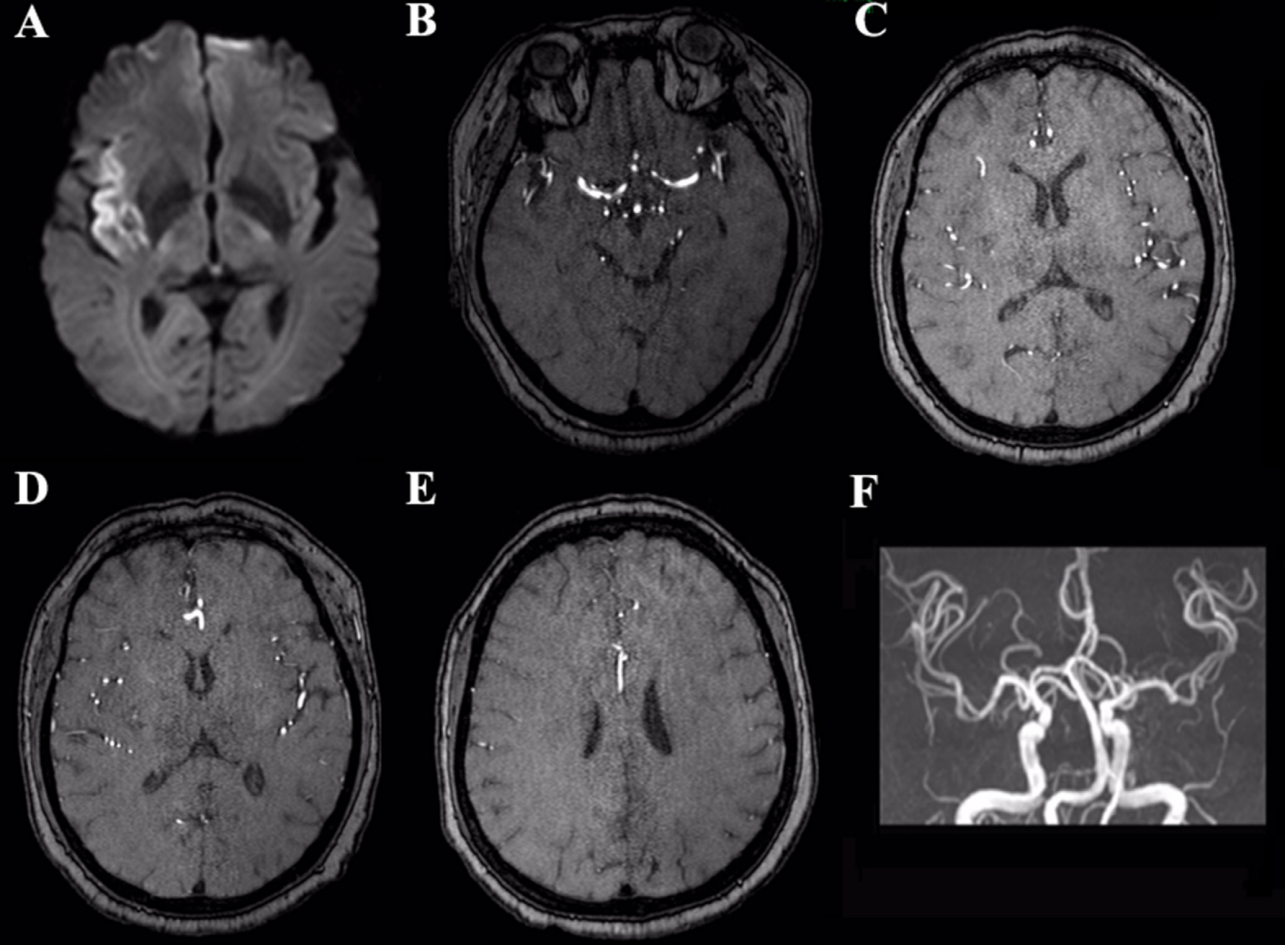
The same case with postoperative MRI imagings (A–F). Postoperative DWI (A) demonstrates the lesion mid enlargement. Sylvian collateral (B, C) adequate. Leptomeningeal collateral (D, E) adequate. 3D-TOF MRA (F) shows right MCA normal.
